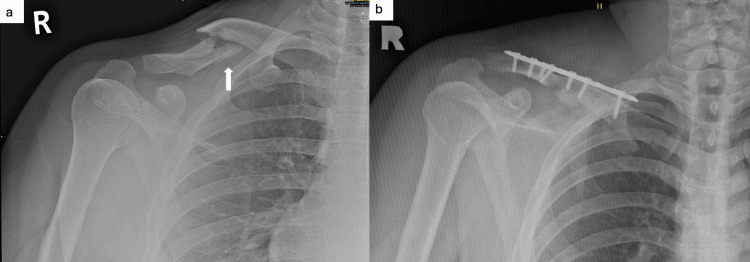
Plain radiograph of the right shoulder. (a) X-ray of the right shoulder post-trauma: comminuted fracture (white arrow) over the middle third right clavicle. (b) X-ray of the right shoulder after primary fixation: fracture fixed with a 3.5 mm straight locking plate (LCP).
